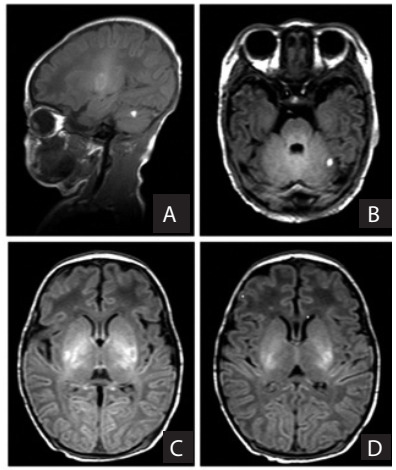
Non-enhanced sagittal (A) and axial (B, C, D) magnetic resonance imaging: abnormally increased signal intensities relating to cerebellar hemorrhage (A) and (B); and bilateral lentiform nucleus and thalamic presence of methemoglobin (C) and (D), as a consequence of hemorrhagic transformation after ischemic injury.
